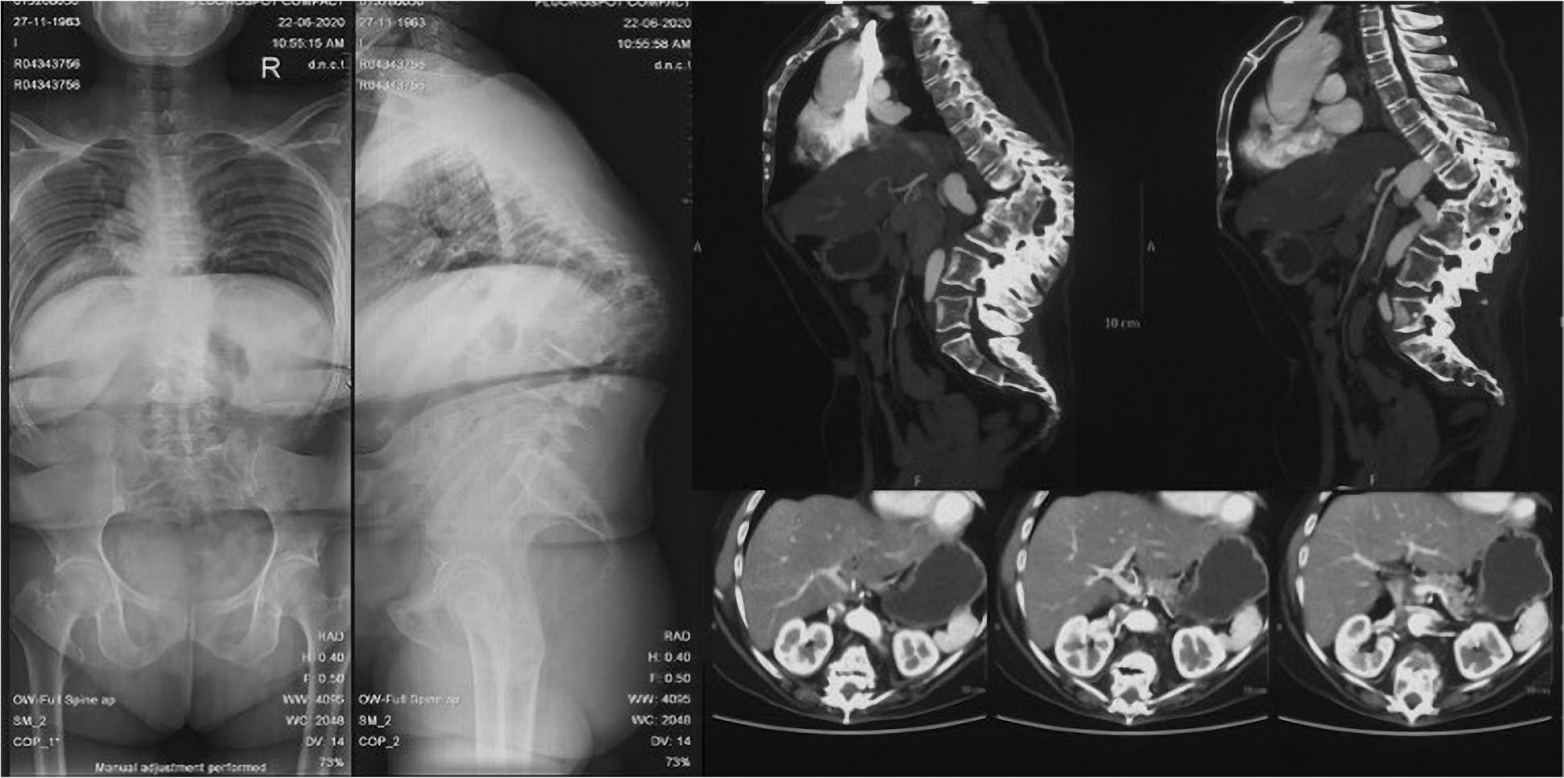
A 57 years old patient presented with numbness of lower limbs. The kyphotic angle of T8-L3 was 96° and SVA was measured 5.0 cm. CT scans showed that the aorta shifted anteromedially to the vertebrae body in at apex vertebral level
